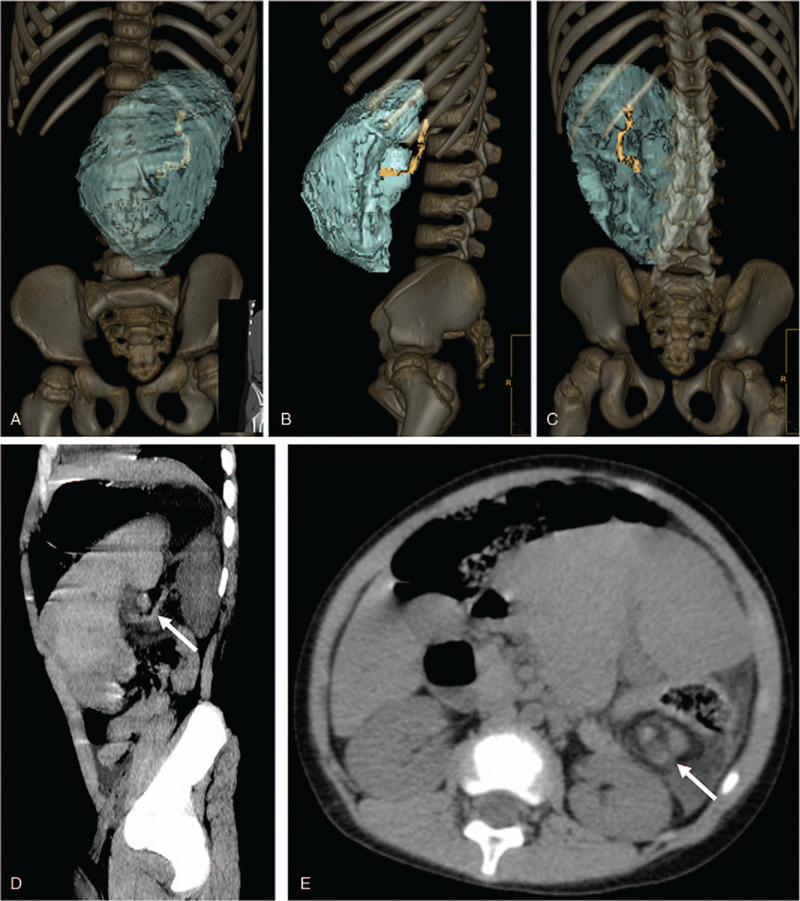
Abdominal CT with 3-dimensional reconstruction demonstrates an ectopic spleen, displaced in the left abdominal cavity. Abdominal CT with 3-dimensional reconstruction (A, B, and C) shows the spleen is enlarged, measuring 15 cm × 10 cm × 7 cm, the splenic vascular pedicle is elongated and flexed (colored in yellow). Sagittal plane of the abdominal CT shows that the splenic vascular pedicle is flexed (D, arrow). In the axial plane of the abdominal CT, the splenic pedicle presents a hyperdense and whirl appearance, which suggests that splenic torsion with surrounding fat accumulation is involved in the twisting (E, arrow). CT = computed tomography.
